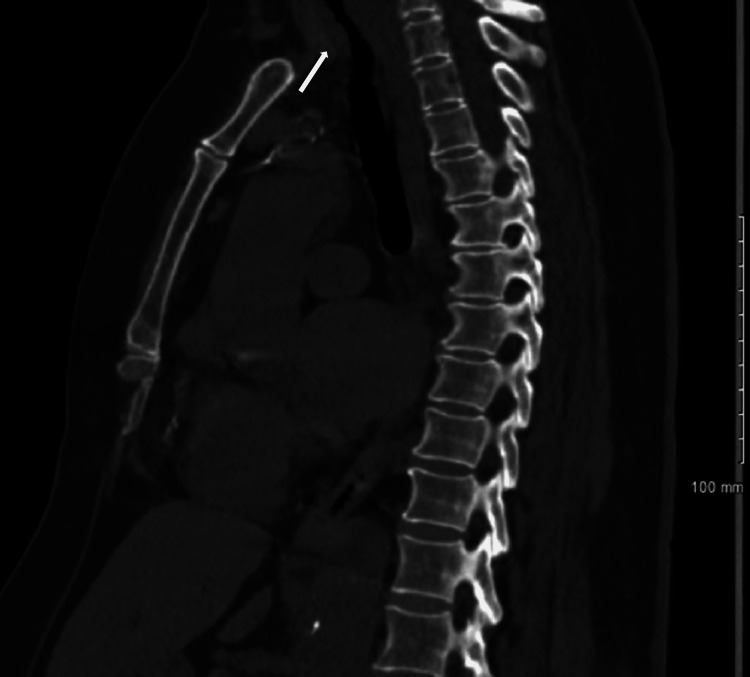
Sagittal view of computed tomography scan of chest without contrast showing tracheal stenosis
